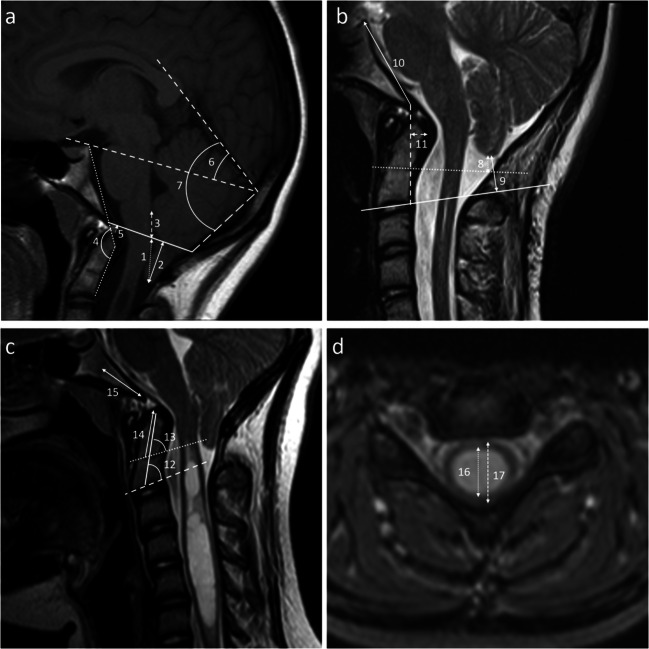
Representative images of the measurements on the T1-weighted (T1W) midsagittal (a), T2-weighted (T2W) midsagittal (b and c), and T2W axial (d) planes. a 1 = vertical distance of the tonsils to the midpoint of the McRae line; 2 = perpendicular distance of the tonsils below the McRae line; 3 = distance from the obex to the midpoint of the McRae line; 4 = angle between the line extending from the top of the dorsum sellae to the basion and the line between the inferodorsal portion of C2 to the most superodorsal part of the dens; 5 = vertical distance of the tip of the dens to the McRae line; 6 = angle between the tentorium cerebelli and a line from the internal occipital protuberance to the tuberculum sellae (Twining’s line); 7 = angle between the tentorium cerebelli and a line from the internal occipital protuberance to the opisthion (slope of the tentorium cerebelli). b 8 = perpendicular distance of the tonsils to the line drawn from the synchondrosis of the C2 vertebra; 9 = perpendicular distance of the tonsils to the line drawn from the end plate of the C2 vertebra; 10 = clivus length (distance from the basion to the posterior clinoid process); 11 = perpendicular distance of the most posterior extent of the odontoid process at the dural interface to a line drawn between the basion and the posterior aspect of the C2 vertebral body (pB-C2 line). c 12 = the angle between the line drawn from the end plate of the C2 vertebra and its intersection with a line drawn from the odontoid tip; 13 = the angle between the line drawn from the synchondrosis of the C2 vertebra and its intersection with a line drawn from the odontoid tip; 14 = length of the odontoid process; 15 = basiocciput length (distance from the basion to the sphenooccipital synchondrosis). d 16 = anteroposterior diameter of the syrinx; 17 = anteroposterior diameter of the spinal cord at the level of the syrinx
